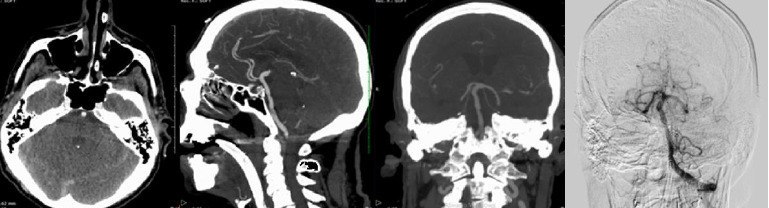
Right panel acute CT-angio (Day 3) and digital angiography (Day 4) showing the atherosclerotic vertebrobasilar system without bleeding source, dissection, or early vasospastic changes.
